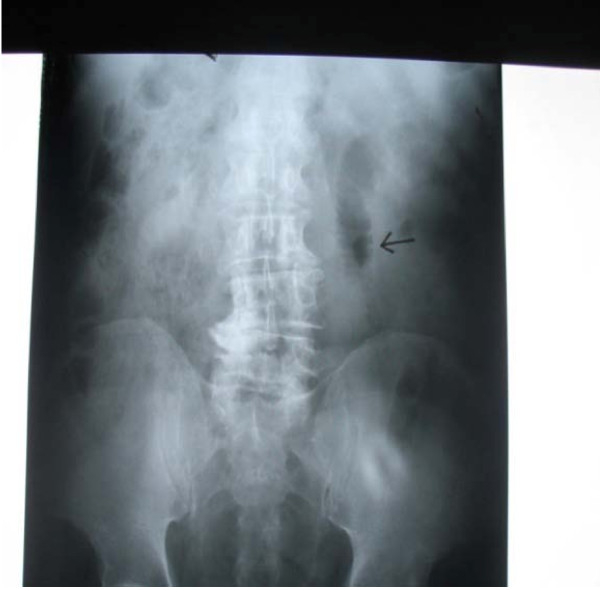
Supine abdominal X-ray with arrow indicating dilated jejunal loop on the left side.
